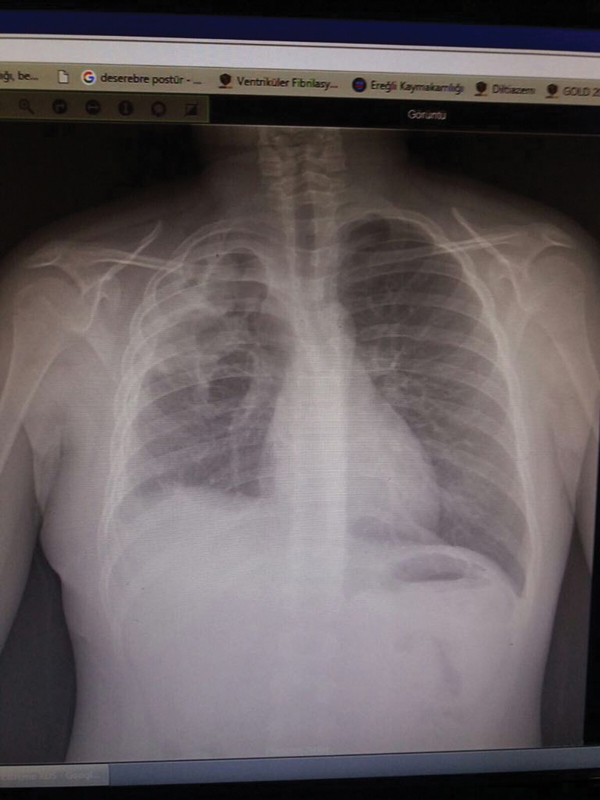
The postoperative chest X-ray showed the expanded right lung parenchyma.
